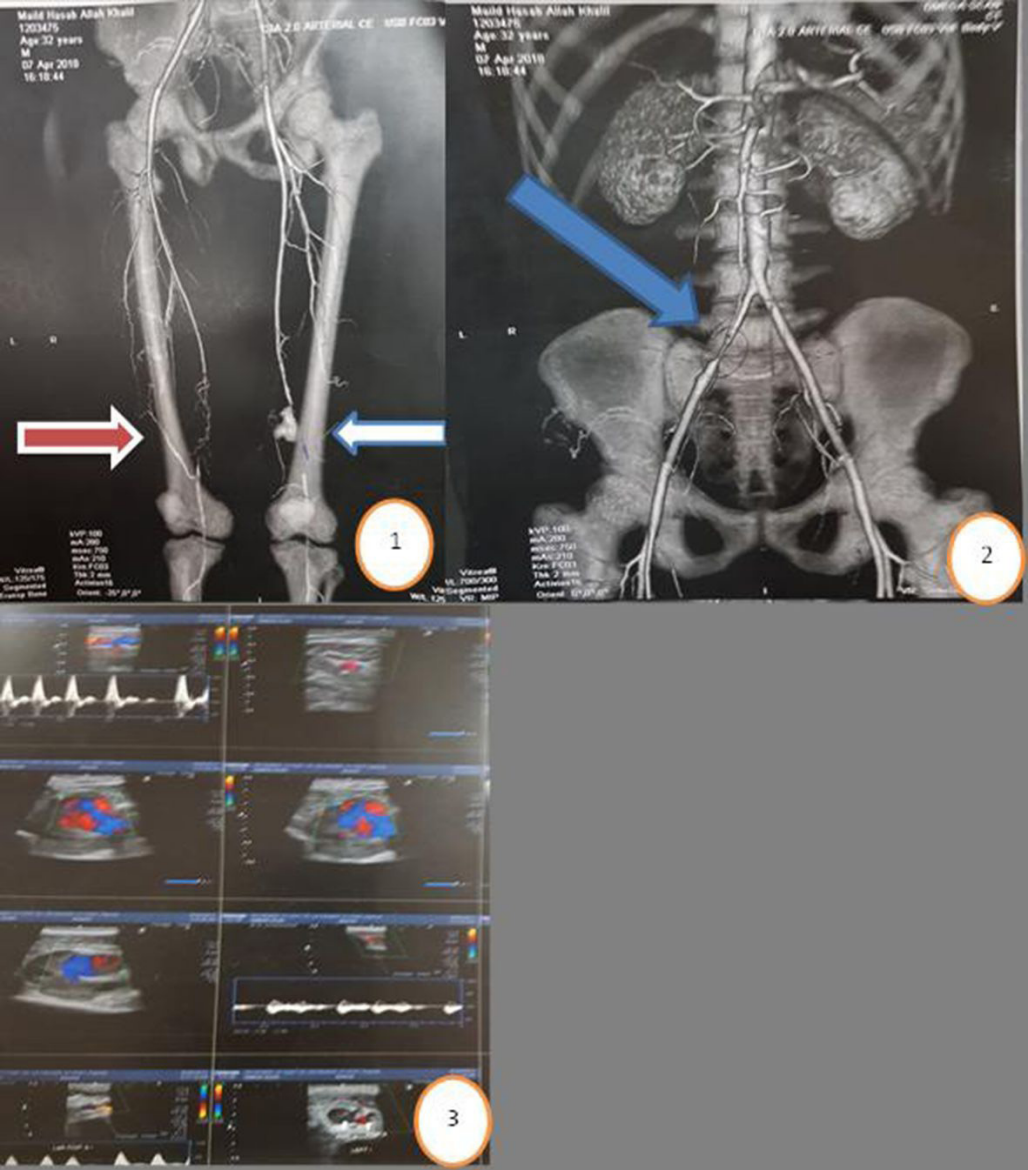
Circle 1: CTA with blue arrow indicating Behçet's pseudoaneurysm of distal left SFA; red arrow indicates associated right SFA block; circle 2: blue arrow indicates associated right iliac occlusion in the same patient, circle 3: Duplex US of left SFA showing pseudoaneurysm.
